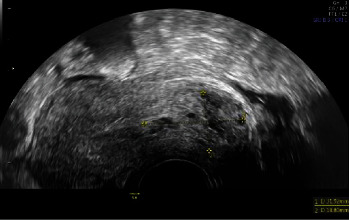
Transvaginal ultrasonographic study of the pelvic-abdomen region shows a retroverted uterus with a biometry of 70 mm x 40 mm. The uterine morphology was regular, and the myometrial ultrasound pattern was homogeneous. The endometrial thickness was 7 mm. The cervical canal was occupied by abundant blood content and clots with a size of 34 mm.
